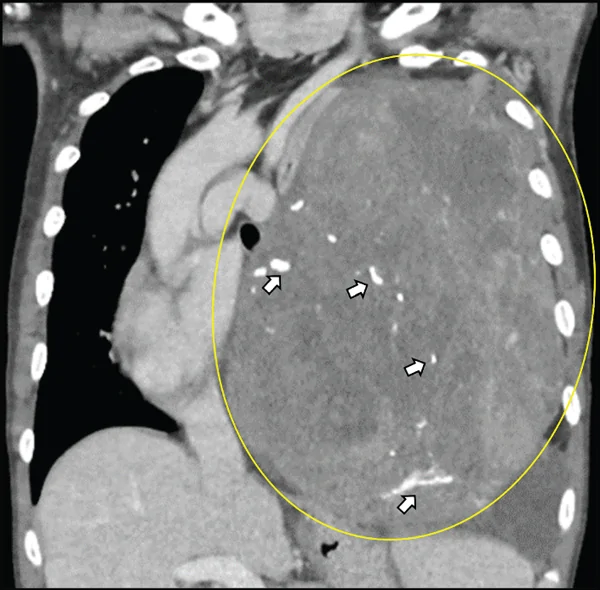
Preoperative coronal chest CT demonstrating a giant heterogeneous pleural‐based mass occupying most of the left hemithorax and causing marked compression of adjacent thoracic structures. The yellow outline delineates the tumor margins, and white arrows highlight internal calcifications.
